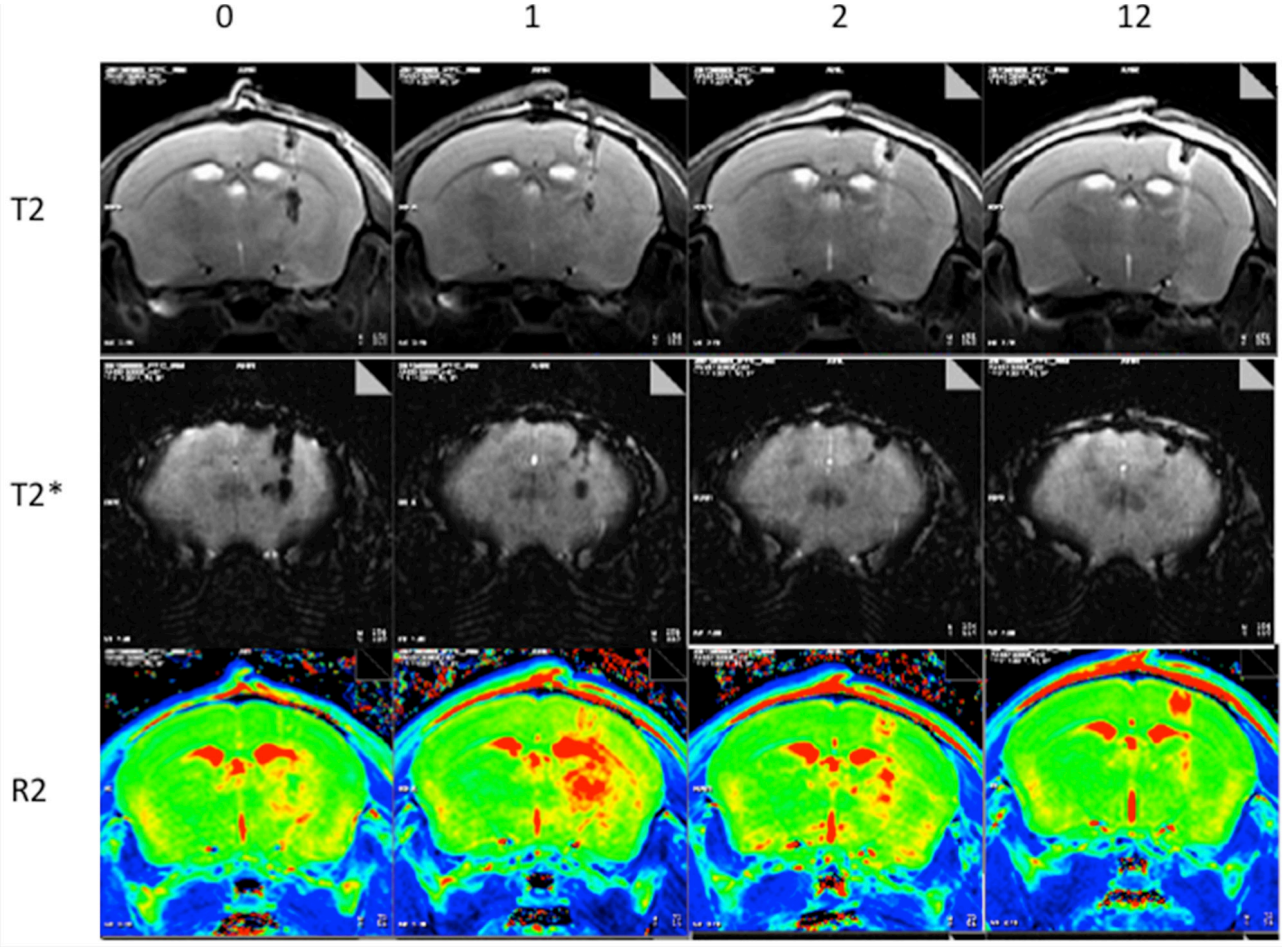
Representative MRI showing longitudinal observations up to 12 hours after Dox-MB infusion (Top: T2 images; Middle: T2* images; Bottom: R2 relaxometry maps).
